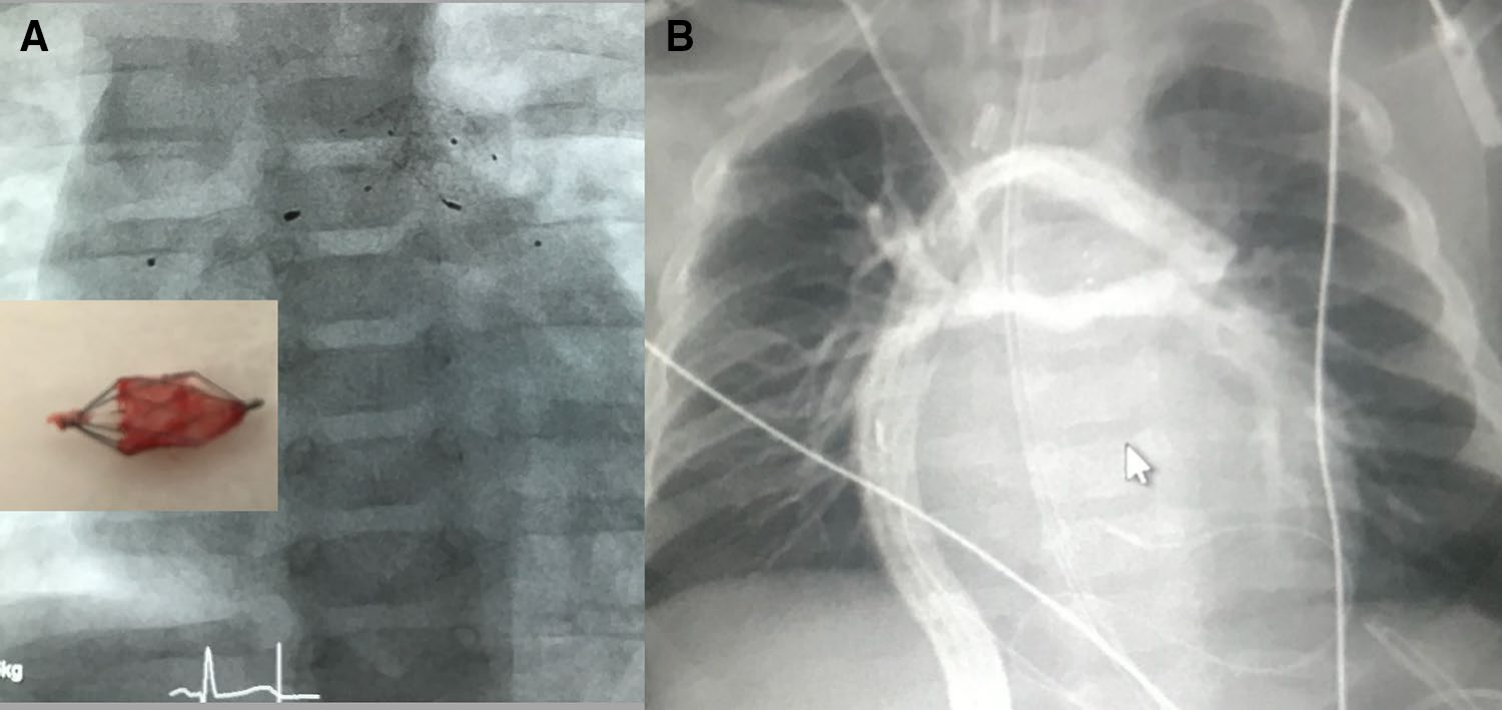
a, b Anterior–posterior x-ray of a ccTGA patient treated with PFRs and ductal stent; shown is also a removed MVP-based flow restrictor (a); figure b shows the contrast-agent-filled pulmonary artery system immediately after comprehensive stage-II surgery
